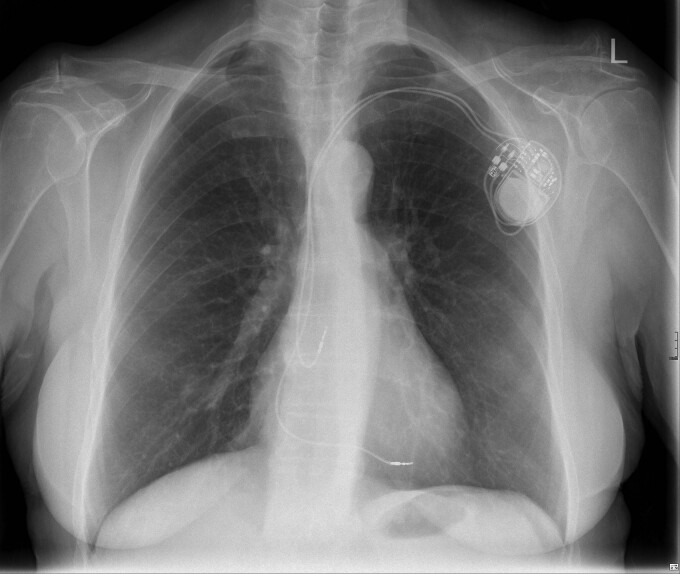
Chest radiograph performed 2 h post procedure.
